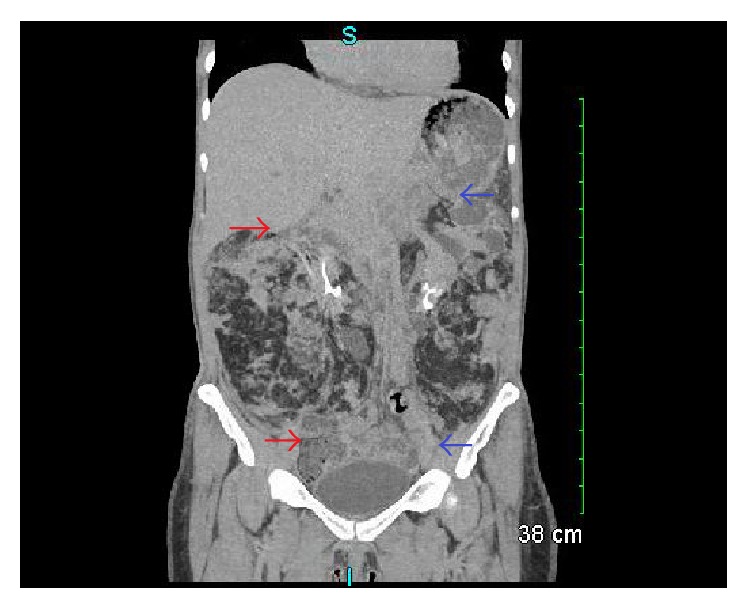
Coronal view of CT urogram showing extensive bilateral AML. Red arrows denote right AML. Blue arrows denote left AML.
